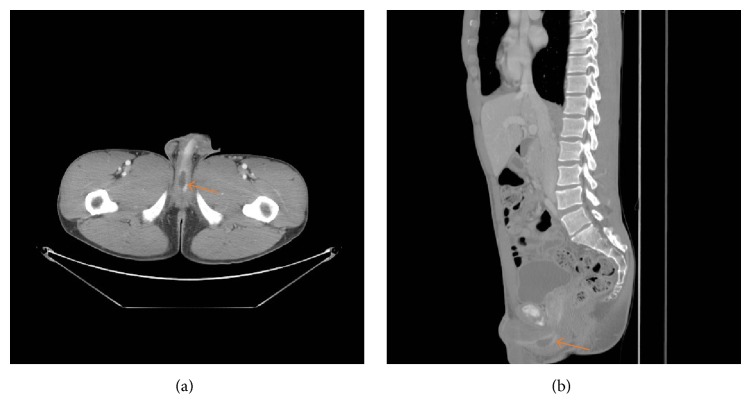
Representative (a) axial and (b) sagittal computed tomography images of the pelvis with contrast demonstrating Cowper's syringocele (arrows).
